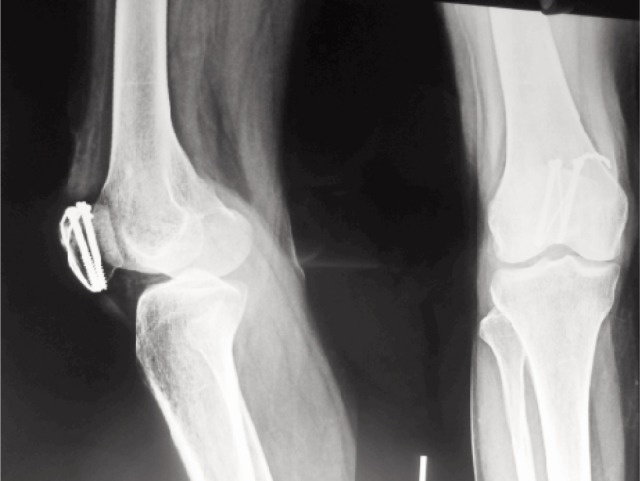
Radiographs demonstrating union at the fracture site (in this patients at 10 weeks) (A) Lateral (B) Anteroposterior radiograph.
